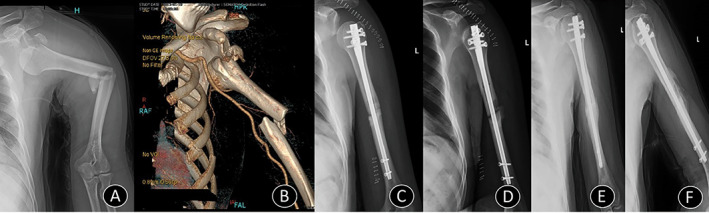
Case 1. (A) A plain radiograph shows dislocation of the shoulder with fractures of the greater tuberosity and humerus shaft. (B) A corresponding three‐dimensional reconstruction of a CT scan. (C) and (D) Immediate postoperative X‐rays showing the fracture fixed with interlocking nail and shoulder reduced, with deltoid palsy. (E) and (F) Six‐month follow‐up X‐ray showing union at the fracture site with reduced shoulder and recovery of deltoid palsy.
